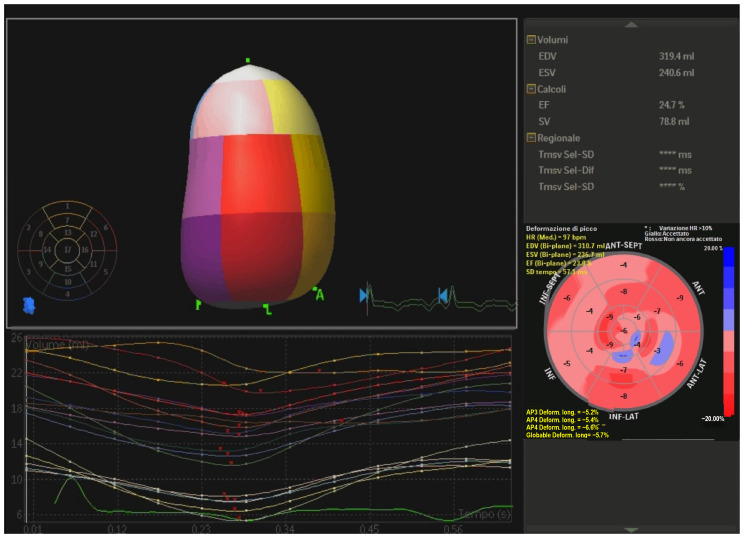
LVEF obtained with 3D echocardiography and GLS measurements in a patient with DCM. In the 3D reconstruction different colors indicates different LV segment. In the Bull’s eye display of GLS intense shades of red indicate optimal GLS values (−20%), while less intense shades, white color and different intensities of blue indicate sub-optimal and pathological GLS values (+20%). EDV = end-diastolic volume; ESV = end-systolic volume; EF = ejection fraction; SV = stroke volume, HR = heart rate, GLS = global longitudinal strain; DCM = dilated cardiomyopathies.
